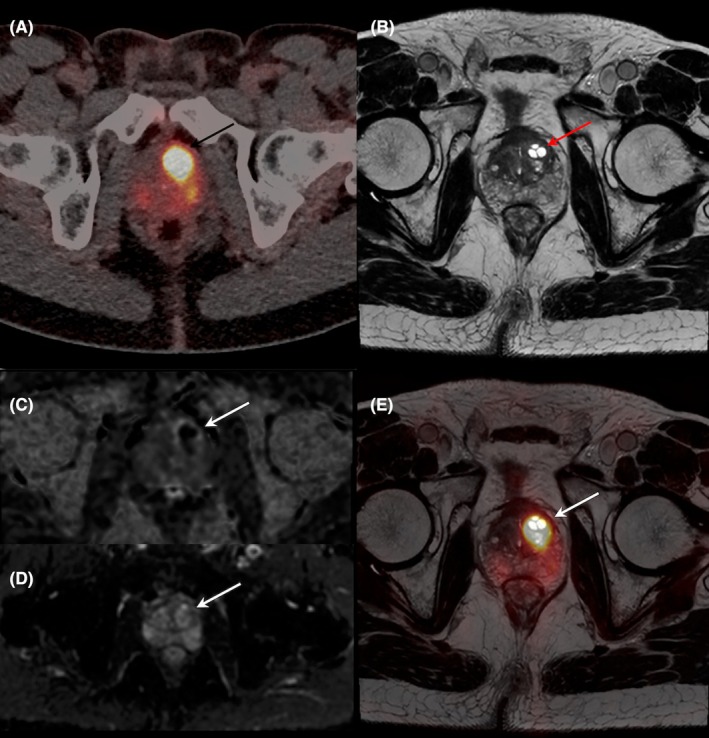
False positive results of [^18^F]DCFPyL PET/CT in a patient presenting with dysuria, without evidence of urinary tract infection, and repeated PSA levels above 20 ng/mL (axial PET/CT (A), T2‐weighted MRI (B), diffusion B sequence (C), ADC sequence (D), PET/MRI fused images (E)). PET/CT revealed two foci of increased uptake of [^18^F]DCFPyL, without any suspicious lesions in the prostate gland on mpMRI. Systematic and targeted transrectal prostate biopsies were performed, and all tissue samples were negative for prostate cancer. Final diagnosis after pathological examination was prostatic adenoma.
